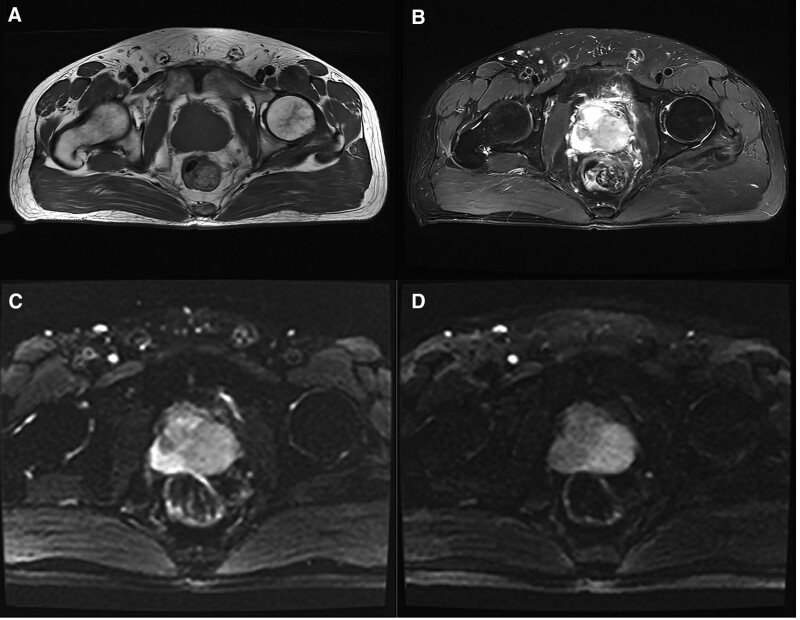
mp-MRI performance: (A) Equal signal displayed on T1WI transverse position; (B) Low signal on compression lipid T2WI; (C) Diffusion limitation on DWI and low signal on ADC; (D) Enhancement MRI showed that the left peripheral zone was a significantly inhomogeneous enhancement. ADC = apparent diffusion coefficient; mp-MRI = multi-parameter magnetic resonance imaging.
